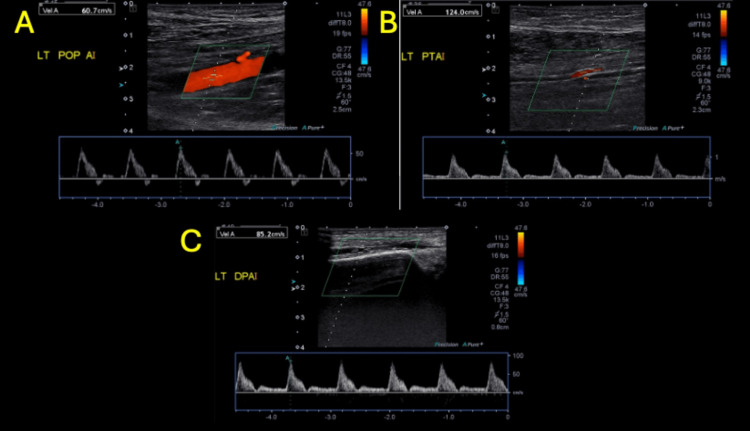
Left lower extremity arterial duplex ultrasound (part 2) Left popliteal artery (LT POP A) with triphasic waveform and peak systolic velocity 74 cm/s (A). Left posterior tibial artery (LT PTA) with monophasic waveform 105 cm/s (B). Left dorsalis pedis artery (LT DPA) with monophasic waveform and peak systolic velocity 120 cm/s (C).
